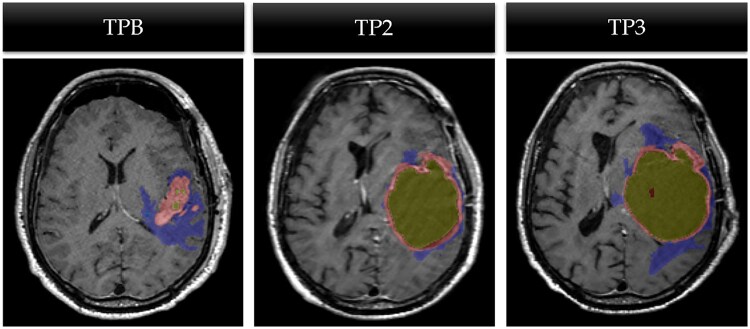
Case example of Neosoma HGG segmentation on axial MR images of a subject with increased resection cavity volume due to accumulation of cerebrospinal fluid showing lack of cystic component at TPB (time point baseline, left panel), with cystic accumulation within the resection cavity at TP2 (time point 2, center panel) and additional cystic enlargement at TP3 (time point 3 right panel). The area of FLAIR-abnormality is shown in blue and its increment over time is most likely secondary to the effects of the increased cyst volume not the tumor recurrence.
